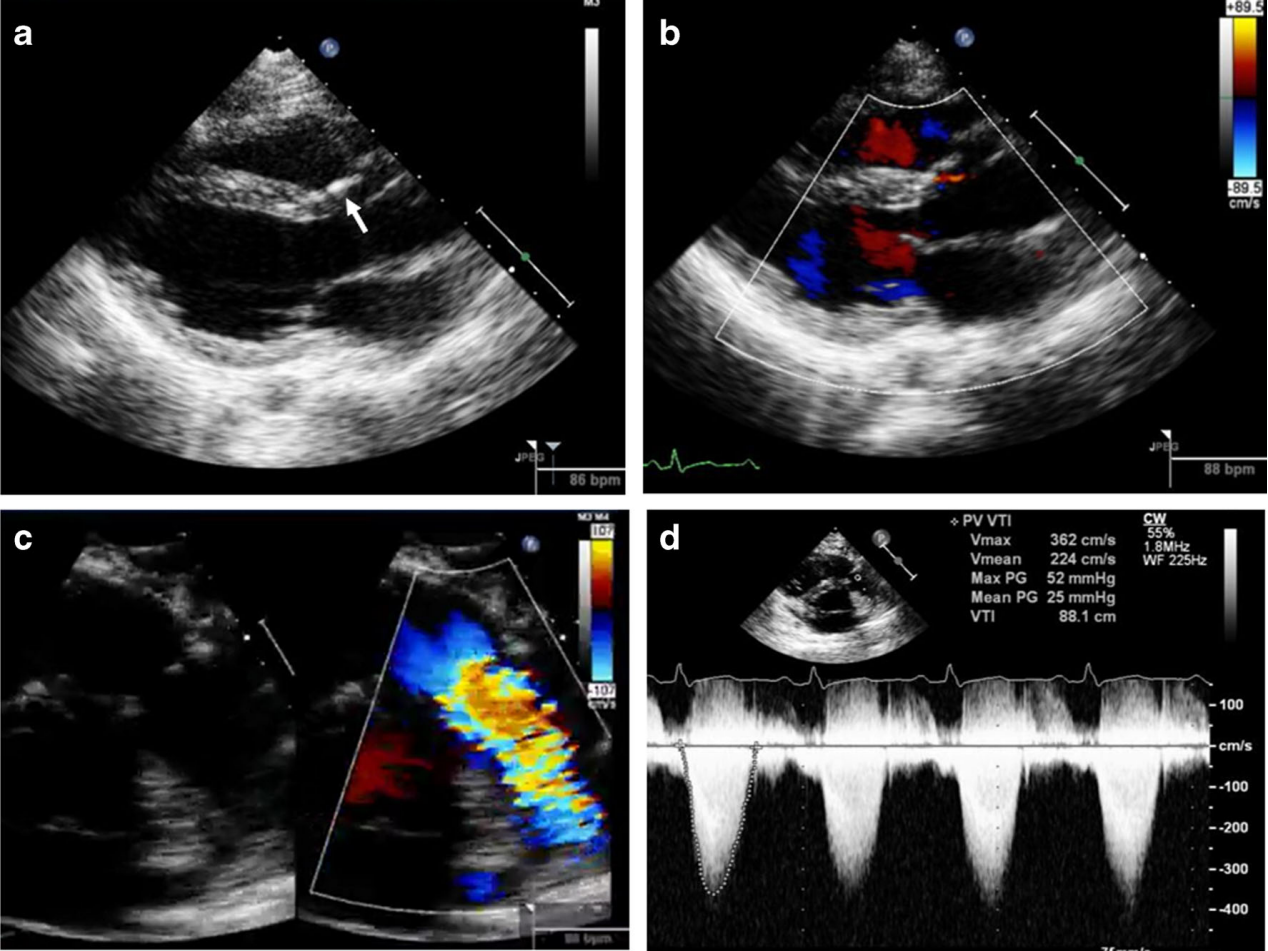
Echocardiographic images in a 24-year-old female G1 at 23 weeks with tetralogy of Fallot and surgeries at ages 3, 6, and 9 with VSD repair, pulmonic valve repair, and then replacement with a homograft. She also had a permanent pacemaker placement at age 14. NYHA class was II (able to walk two to three blocks at ground level and up 10 stairs). Images show the VSD patch in a (*white arrow*). There was no residual VSD flow and there was trace aortic regurgitation (b). Turbulent color Doppler flow across pulmonic prosthesis (c) with peak and mean gradients of 32 and 25 mm Hg, respectively, is shown (d). Patient is being closely followed in the OB cardiology clinic. The RV was mildly enlarged with paradoxical IVS motion and mildly reduced with RV systolic function. RV outflow tract was mildly dilated and there was intrinsic atrial rhythm (86–88 bpm) without RV pacing
